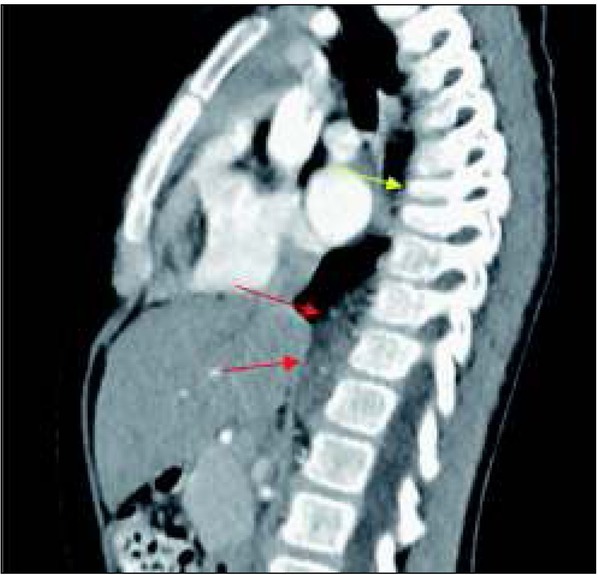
Obraz TK klatki piersiowej z kontrastem z uwidocznionym guzem okolicy przykręgosłupowej (czerwone strzałki) i złamaniem kompresyjnym trzonów kręgów (żółta strzałka). Fig. 2. Contrast computed tomography scan showing a paravertebral tumor (red arrows) and compression fractures of vertebral bodies (yellow arrow).
